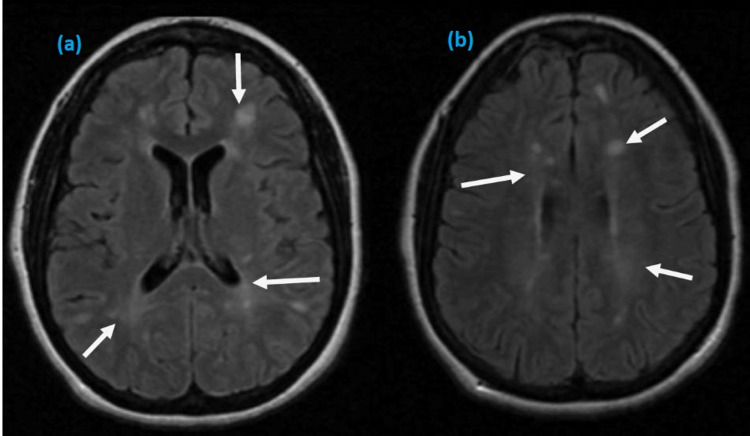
Axial T2 MRI images showing hyperintense signals in both hemispheres (a, b). MRI: Magnetic resonance imaging
